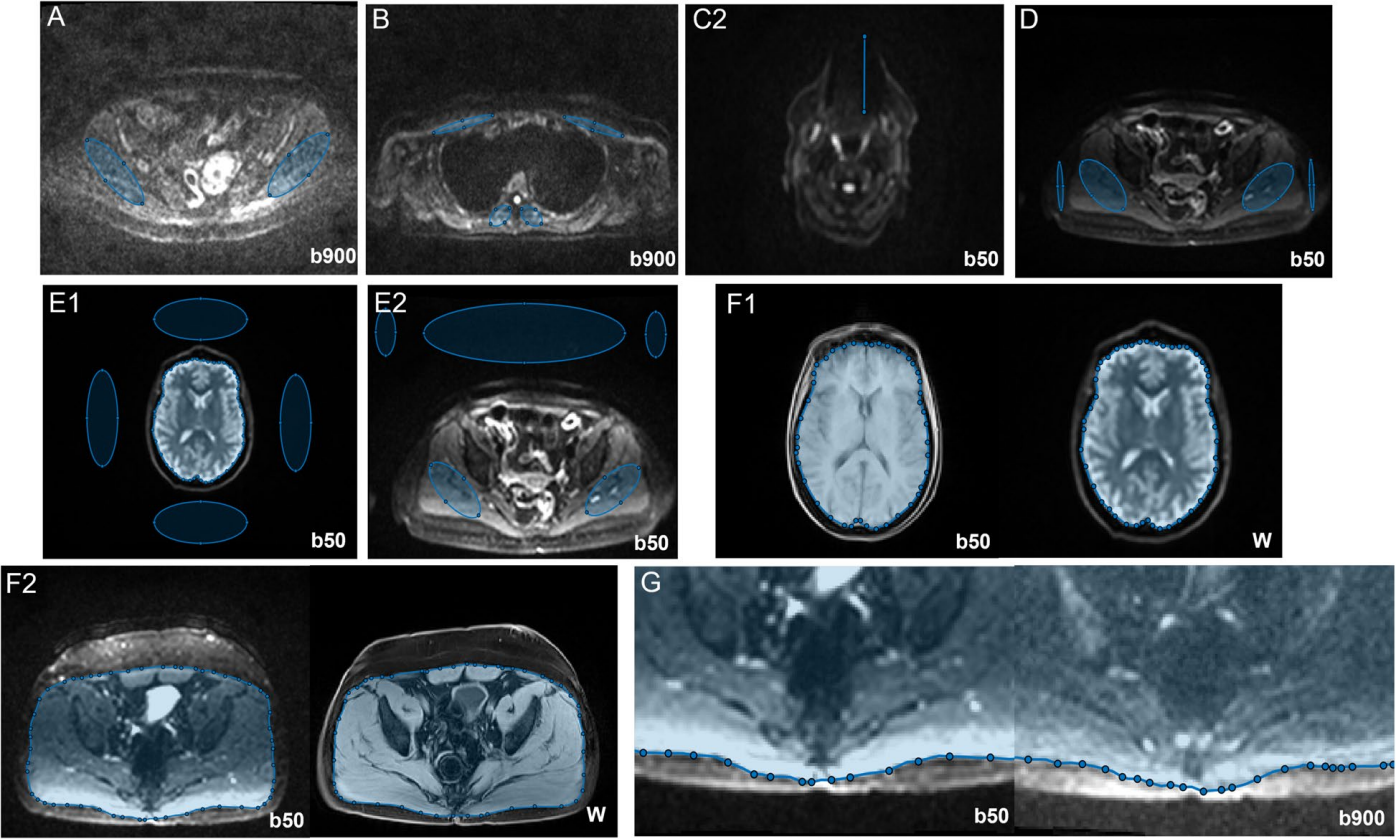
Examples of the method for calculating the quantitative metrics. The metrics for each artefact/image quality issue are defined in Table 2. The size of the ROIs varied between patients in accordance with anatomical differences. Metrics: A—signal to noise ratio; B—anterior thoracic signal loss; C2—susceptibility artefact, length; D—fat suppression; E1—ghosting (brain); E2—ghosting (pelvis); F1—geometric distortion (brain); F2—geometric distortion (pelvis); G—eddy current distortion. Image series: b50—DWI with *b*-value = 50 smm^−2^; b900—DWI with *b*-value = 900 smm^−2^; W—Dixon water image
